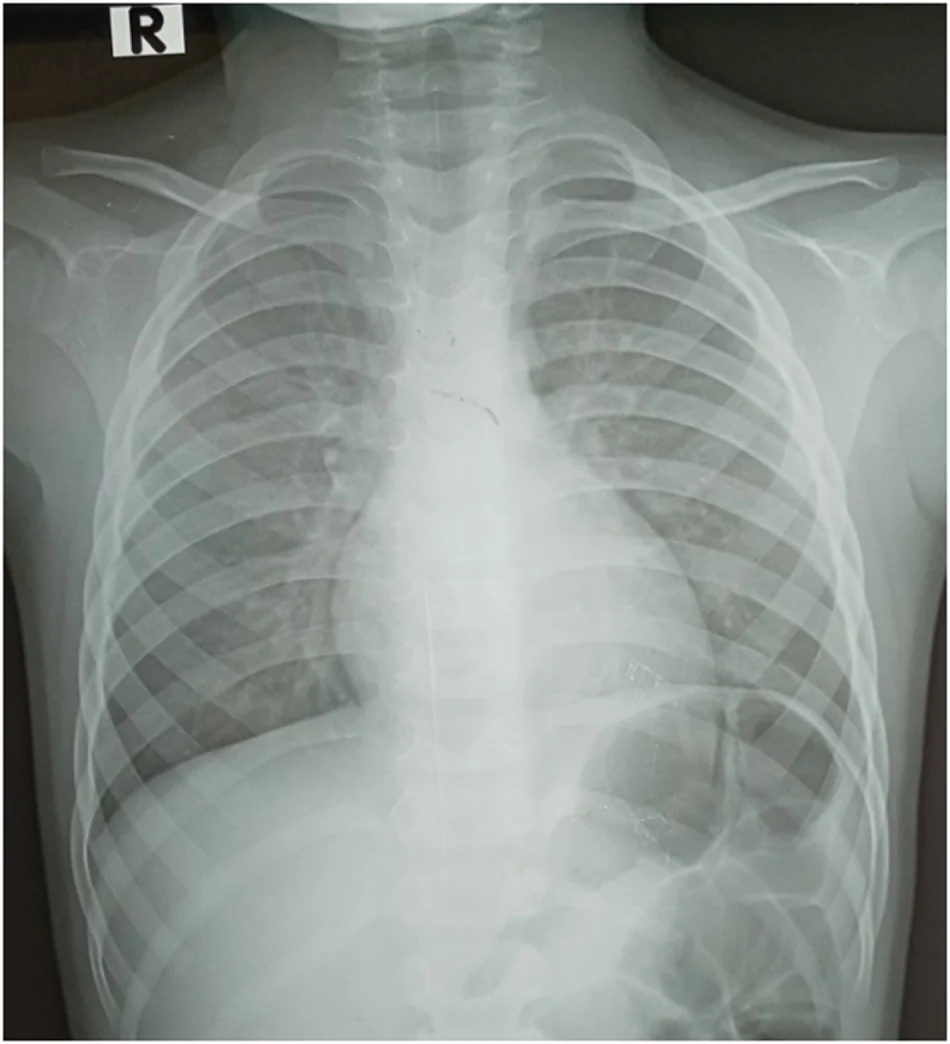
Chest X-ray. Note normal cardiac orientation excluding thoracic situs inversus.
